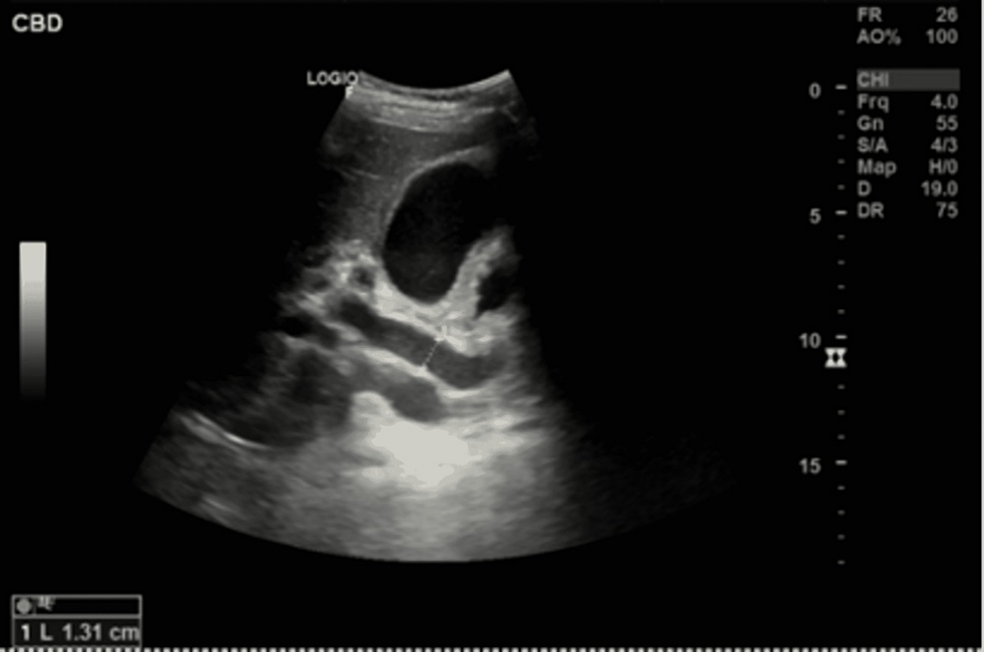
Abdominal ultrasonography showing dilated common bile duct 13m
